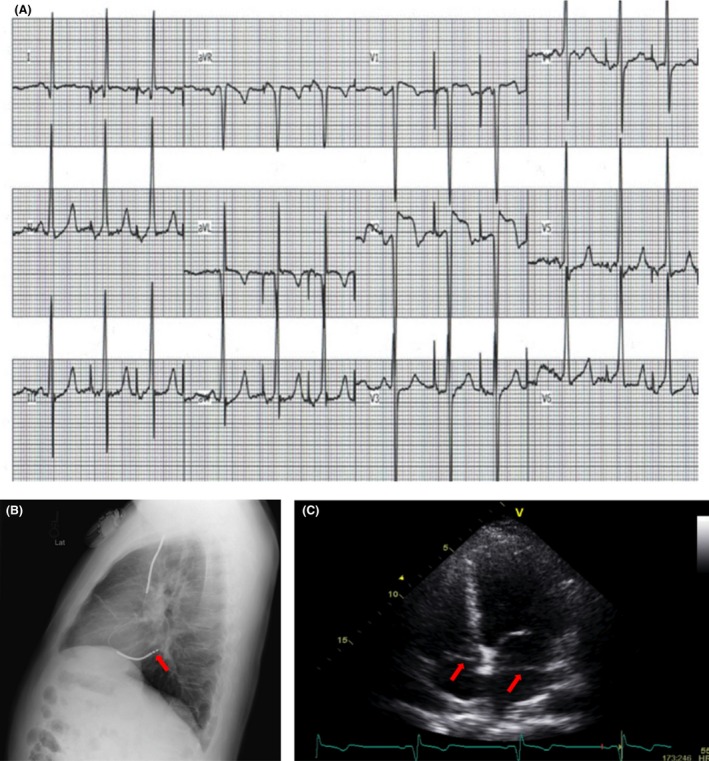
Pacing at maximum output with left atrial capture. The first beat was not paced with normal sinus beat morphology (A). Chest X‐ray with lateral projection demonstrating ICD lead position (arrow) suggestive for misplacement within the coronary sinus or left atrium (B). 4‐chamber view of transthoracic echocardiography showing the ICD lead creating a shadow in the left atrium (arrow) (C).
